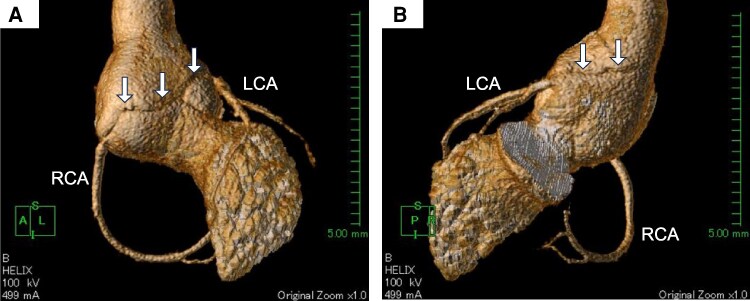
Cardiac-gated 3D computed tomography. 3D reconstruction computed tomography images showed a spiral dissection (arrow) extending from the right coronary ostium to just above the left coronary ostium and reaching the vicinity of the sinotubular junction (*A* and *B*). LCA, left coronary artery; RCA, right coronary artery.
